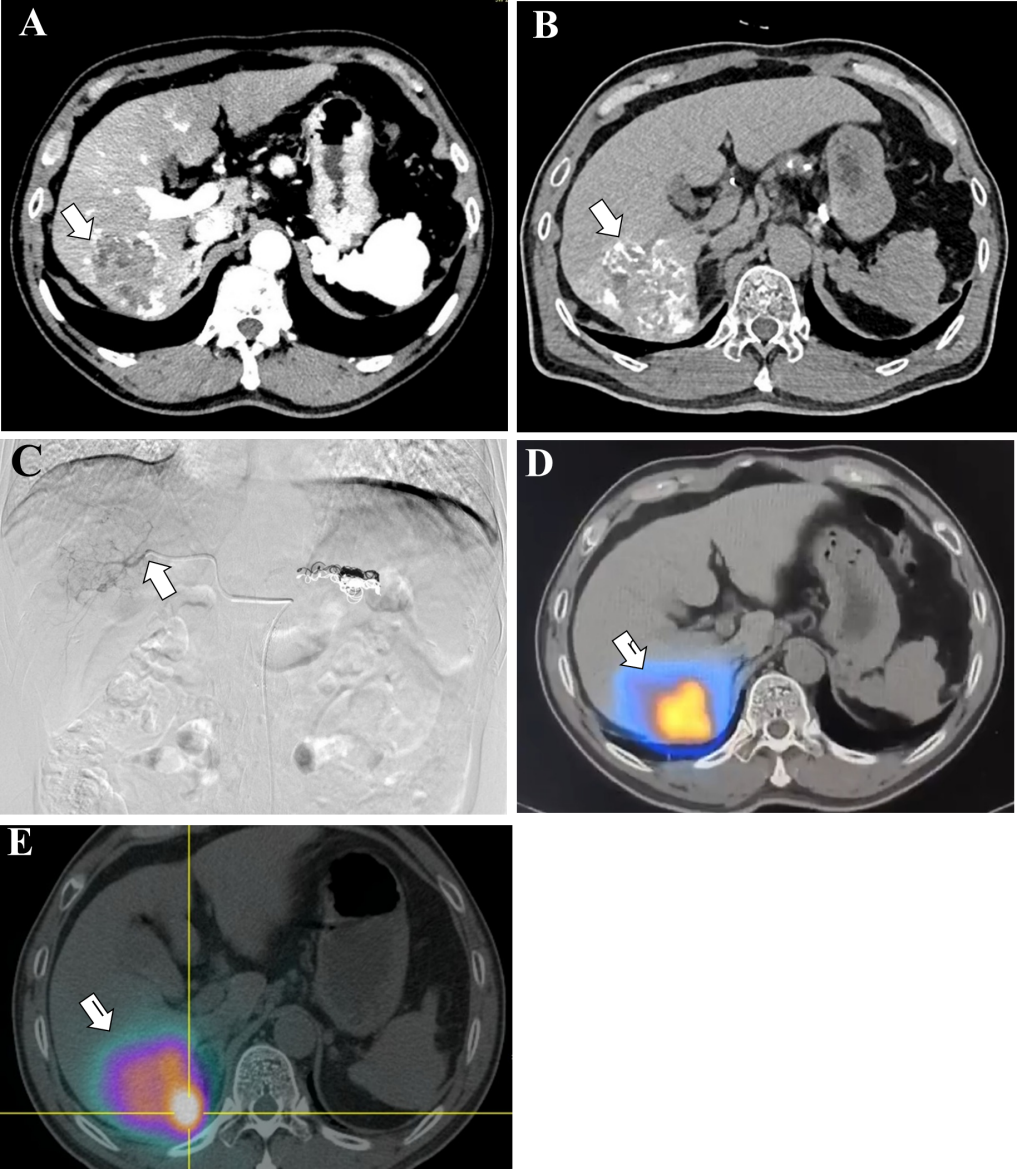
Post-procedural dose verification. Images in a 56-year-old man with hepatocellular carcinoma demonstrate that the intratumoral distribution of ^90^Y microspheres was essentially consistent with the pretreatment ^99m^Tc-MAA prediction. (A) Axial contrast-enhanced CT image (arterial phase) shows an arterially enhancing lesion (arrow) in the right hepatic lobe, corresponding to hepatocellular carcinoma. (B) Axial Angio-CT image obtained after superselective catheterization of the posterior branch of the right hepatic artery again shows the arterially enhancing lesion (arrow). (C) Selective angiogram of the posterior branch of the right hepatic artery shows appropriate catheter position (arrow) for ^99m^Tc-MAA delivery. (D) Coregistered ^99m^Tc-MAA SPECT/CT and CT image shows essentially complete tumoral distribution of the ^99m^Tc-MAA (arrow). (E) Coregistered posttherapy ^90^Y bremsstrahlung and pretherapy CT image similarly demonstrates similar intratumoral distribution of ^90^Y microspheres (arrow).
